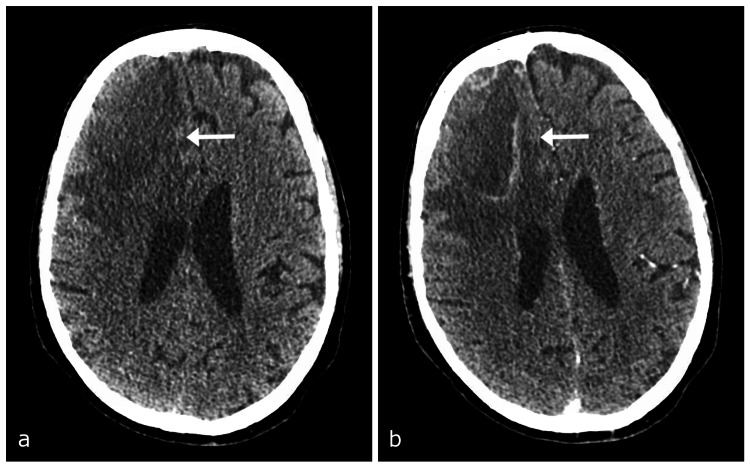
Initial imaging demonstrating a ring-enhancing lesion with surrounding vasogenic edema and mass effect in the right frontal lobe. (a) CT head without contrast and (b) with contrast. CT, computed tomography
